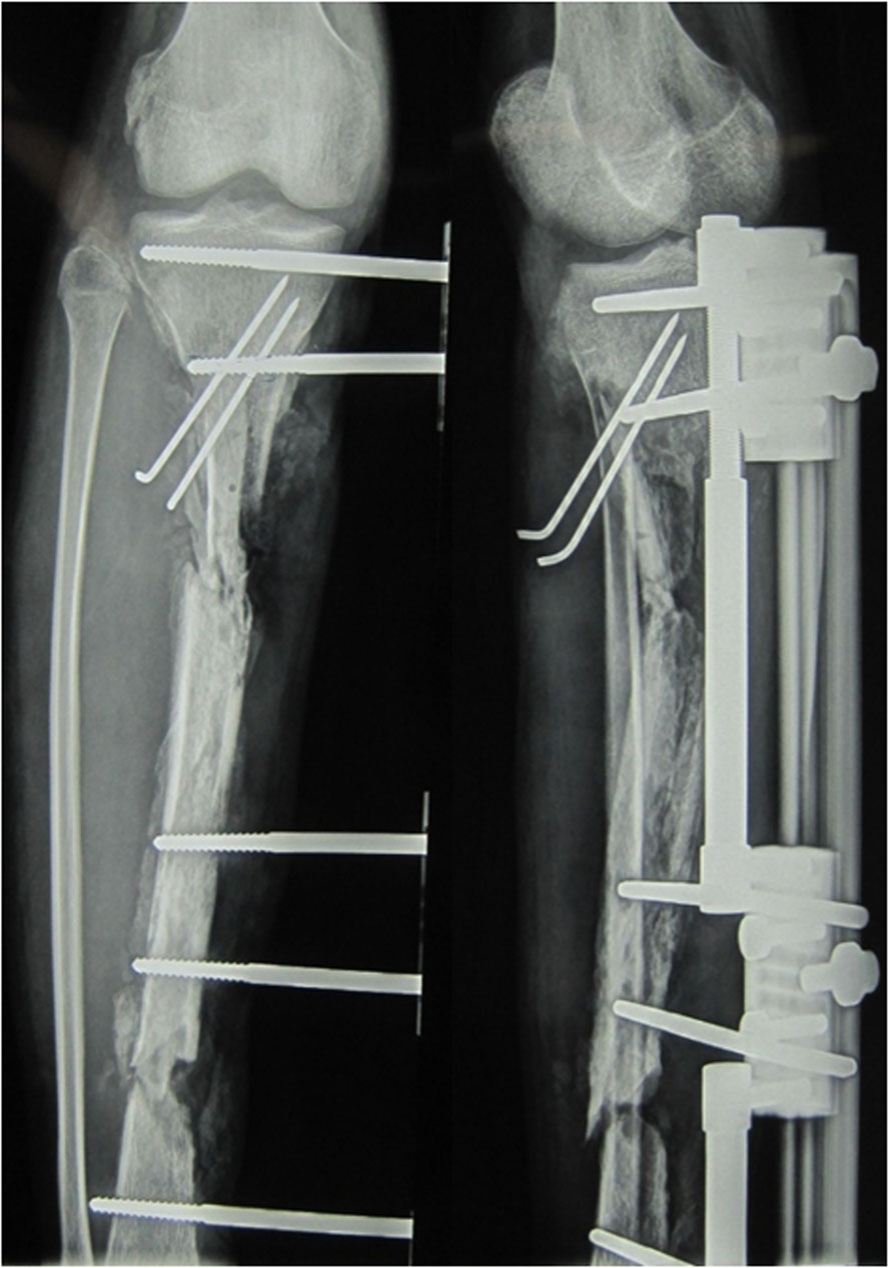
Preoperative X-ray on the day of admission at The Second People’s Hospital of Yunnan demonstrating the necrotic bone
